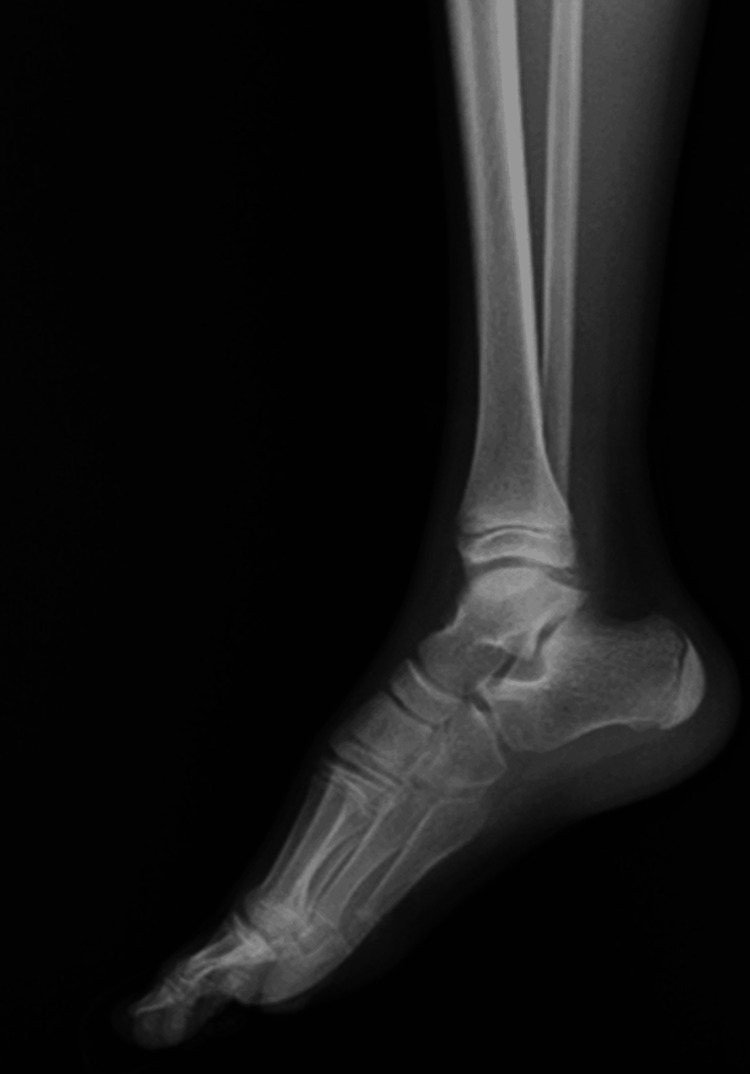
Lateral Right Foot X-ray Lateral right foot X-ray showing no abnormalities.
